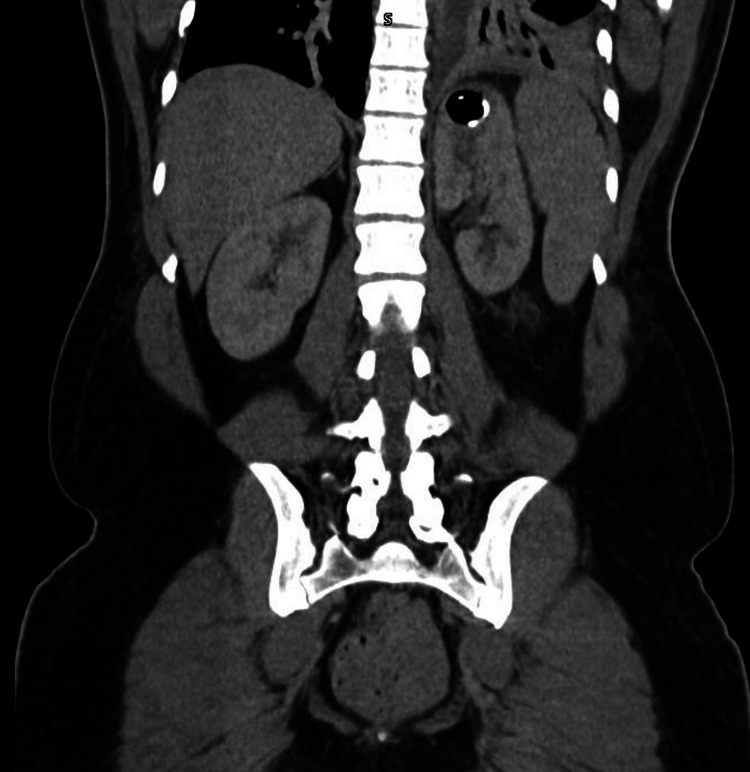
CT of the abdomen/pelvis with contrast, coronal view, showing decompression of the abscess with the stent in place.
